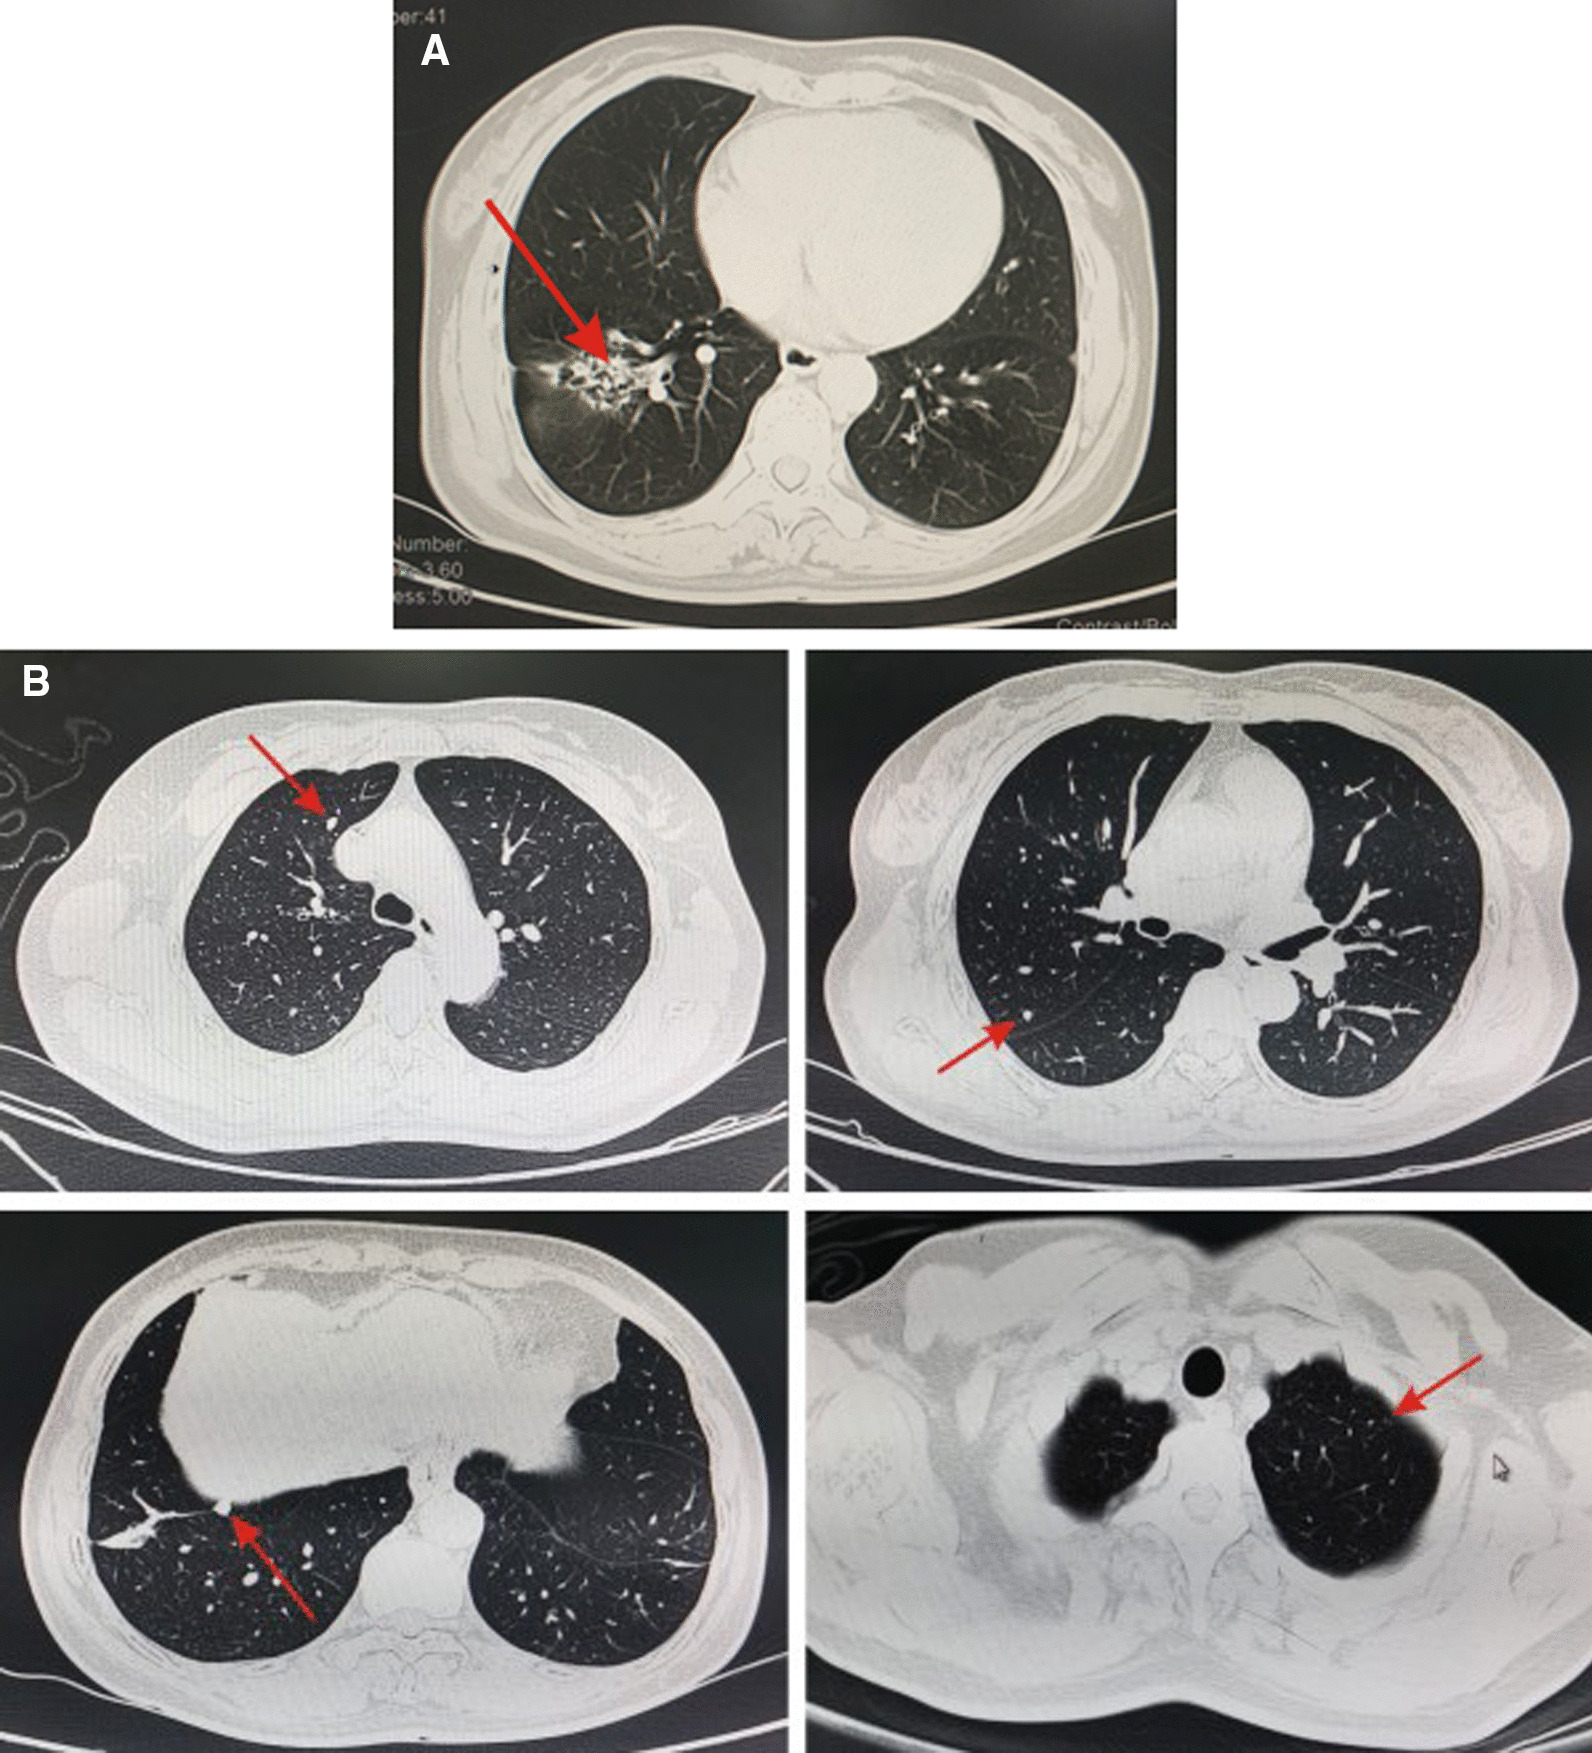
The chest CT scan showed the space-occupying lesion in the inferior lobe of the right lung (arrows) (A) and multiple small nodules in both lungs (arrows) (B)
